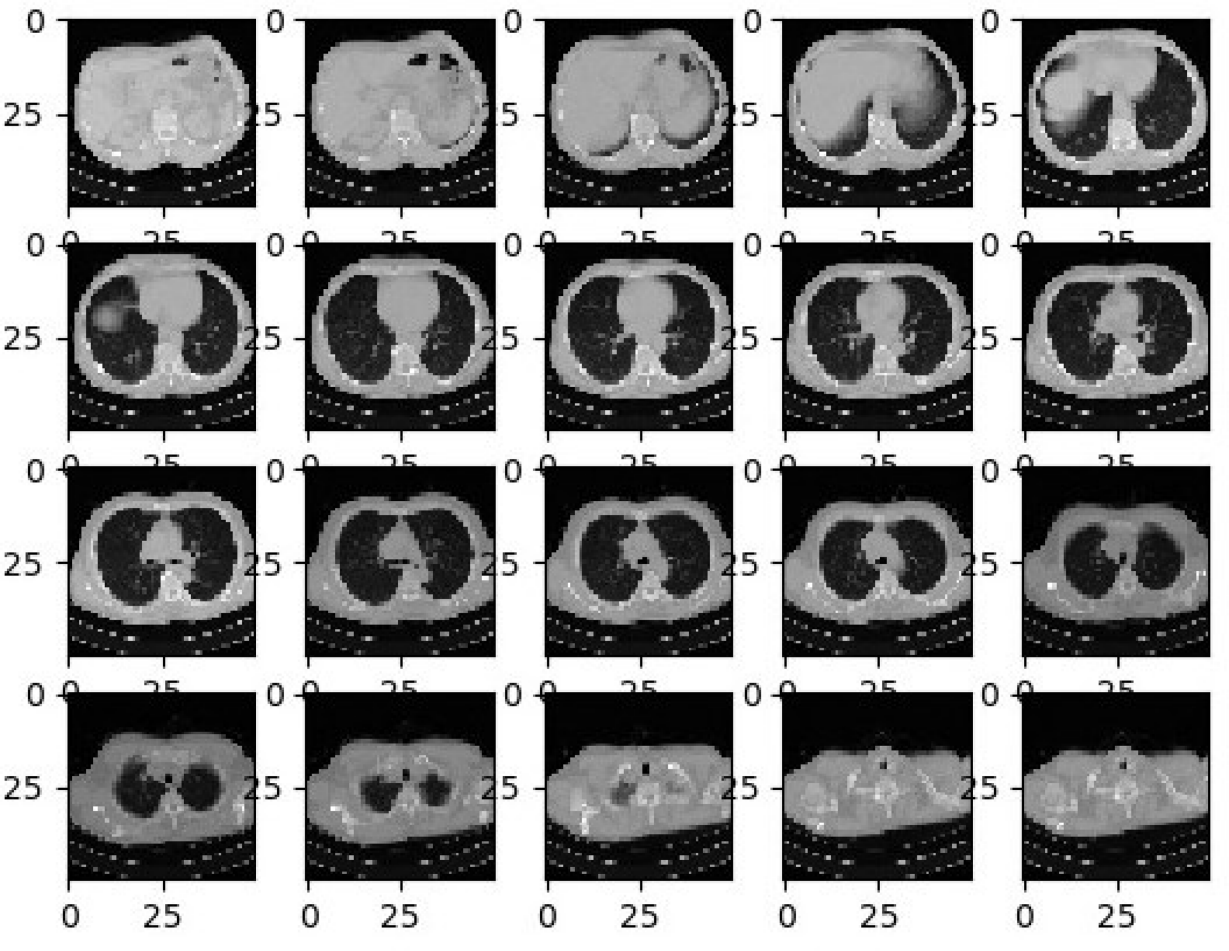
Sample CT-Lung Images after Augmentation Process.
